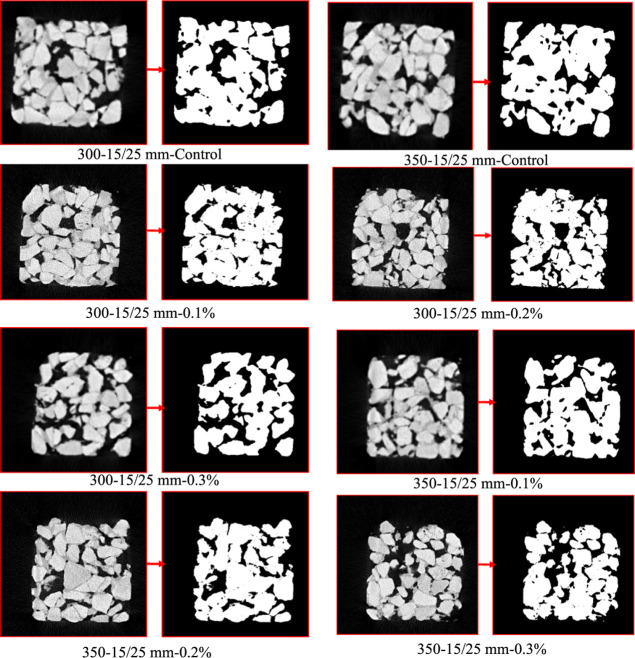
Raw and binary CT images of pervious mixes.
